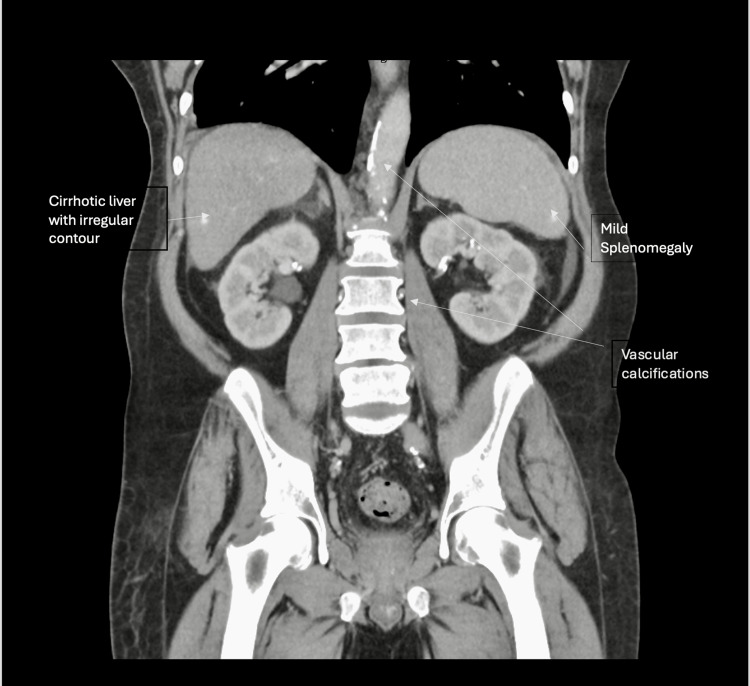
Contrast-enhanced computed tomography of the abdomen demonstrating chronic liver disease and splenomegaly Coronal contrast-enhanced CT image showing hepatomegaly with irregular liver contour, mild splenomegaly, and extensive vascular calcifications involving the abdominal aorta and major abdominal vessels. No portal or splenic vein thrombosis was evident on imaging.
